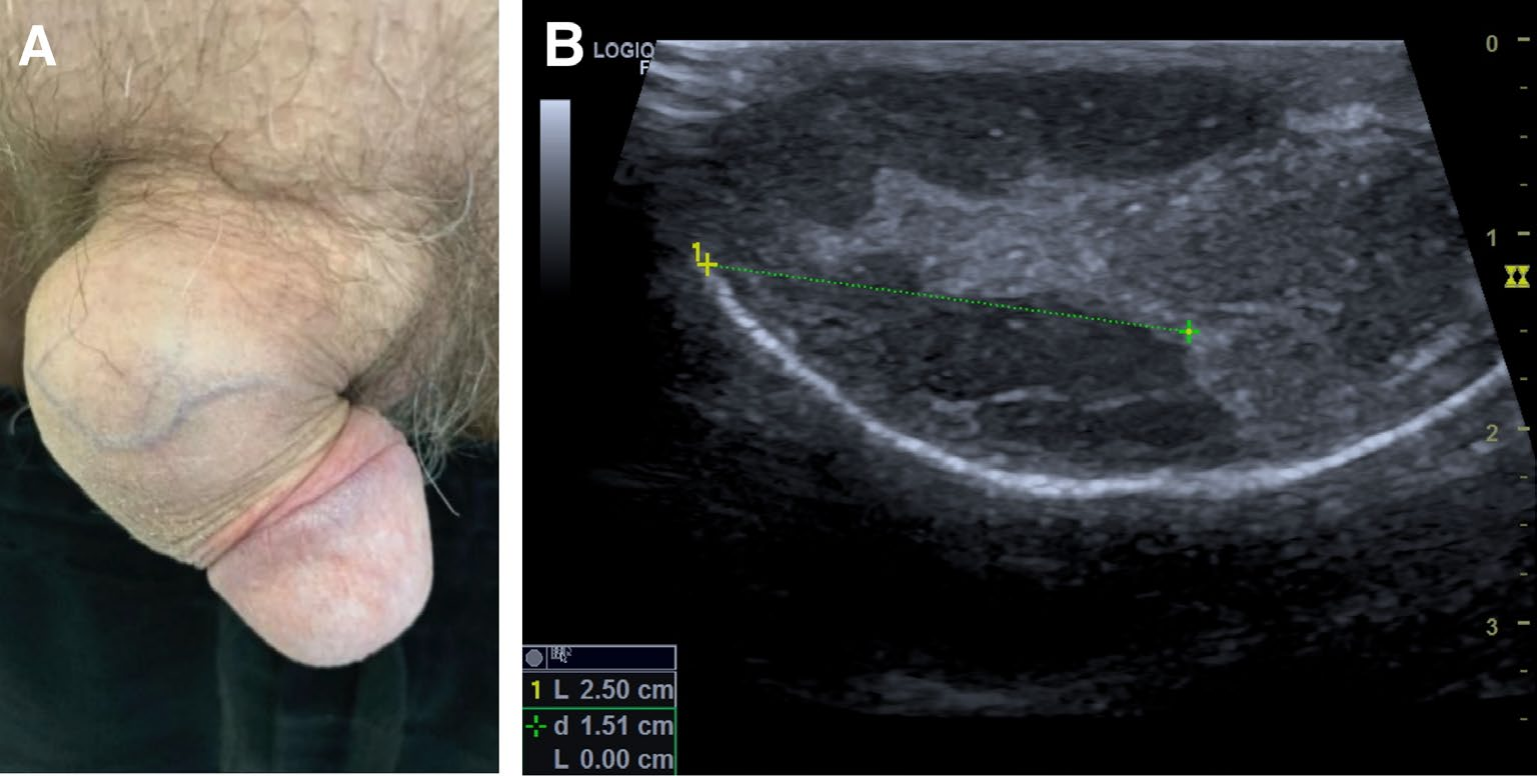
First case. (A) dorsal‐lateral subcutaneous penile granuloma in the middle third of the penis, (B) ultrasound image.
